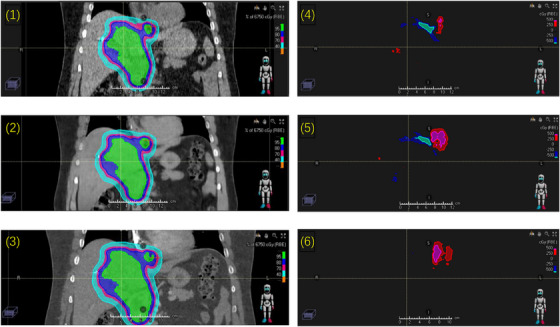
A comparison of the dose plans generated using different CTs and the differences. 1) The dose plan generated using the true NCECT (the planning CT, without contrast). 2) The dose plan generated using the generated NCECT. 3) The dose plan generated using the CECT. 4) The difference between 1 and 2. 5) The difference between 1 and 3. 6) The difference between 2 and 3.
